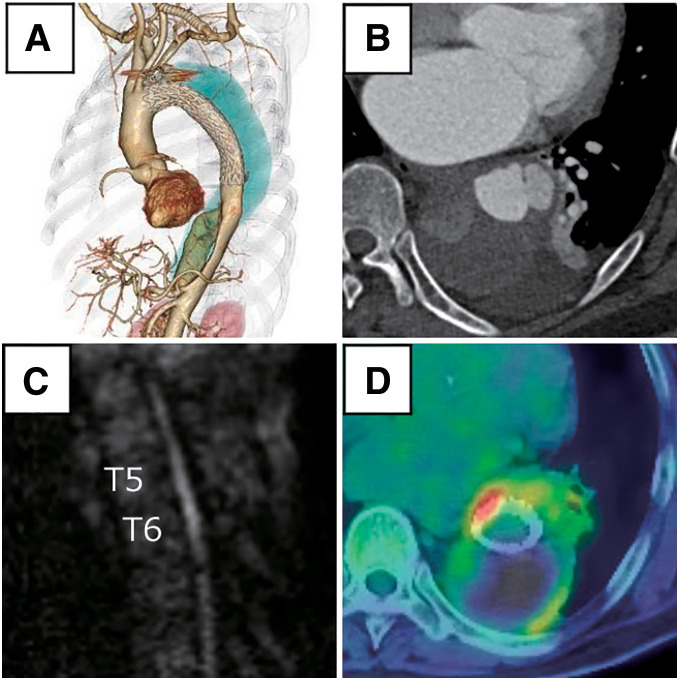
Preoperative imaging findings demonstrating stent graft infection and spinal cord infarction. (A) Preoperative 3-dimensional CT showing an aortic aneurysm. (B) Contrast-enhanced CT revealing an infected saccular aneurysm (77 × 55 mm) at the distal edge of the stent graft. (C) Diffusion-weighted magnetic resonance imaging showing high signal intensity in the spinal cord at the T5–T6 level, consistent with acute spinal cord infarction. (D) FDG-PET/CT demonstrating intense uptake along the stent graft and surrounding aneurysmal wall, indicative of infection. CT: computed tomography; FDG: ^18^F-fluorodeoxyglucose; PET: positron emission tomography
